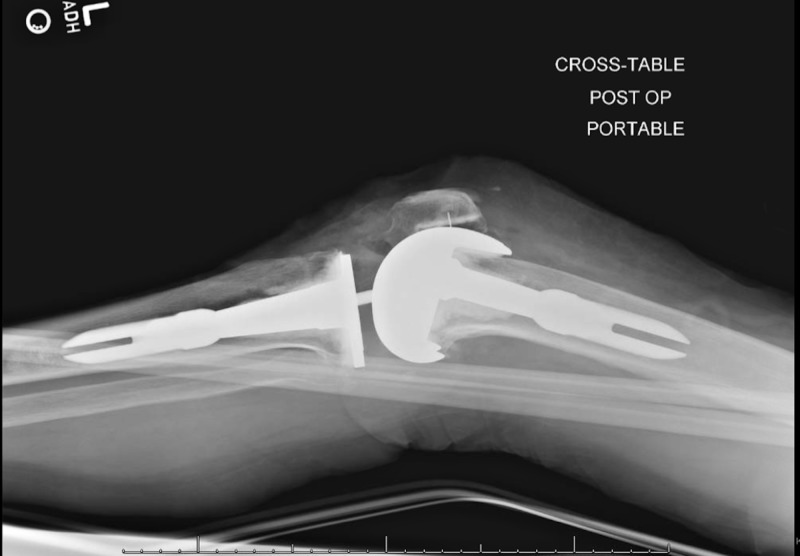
Postoperative lateral radiograph confirming proper placement of left revision total knee arthroplasty (TKA) components
